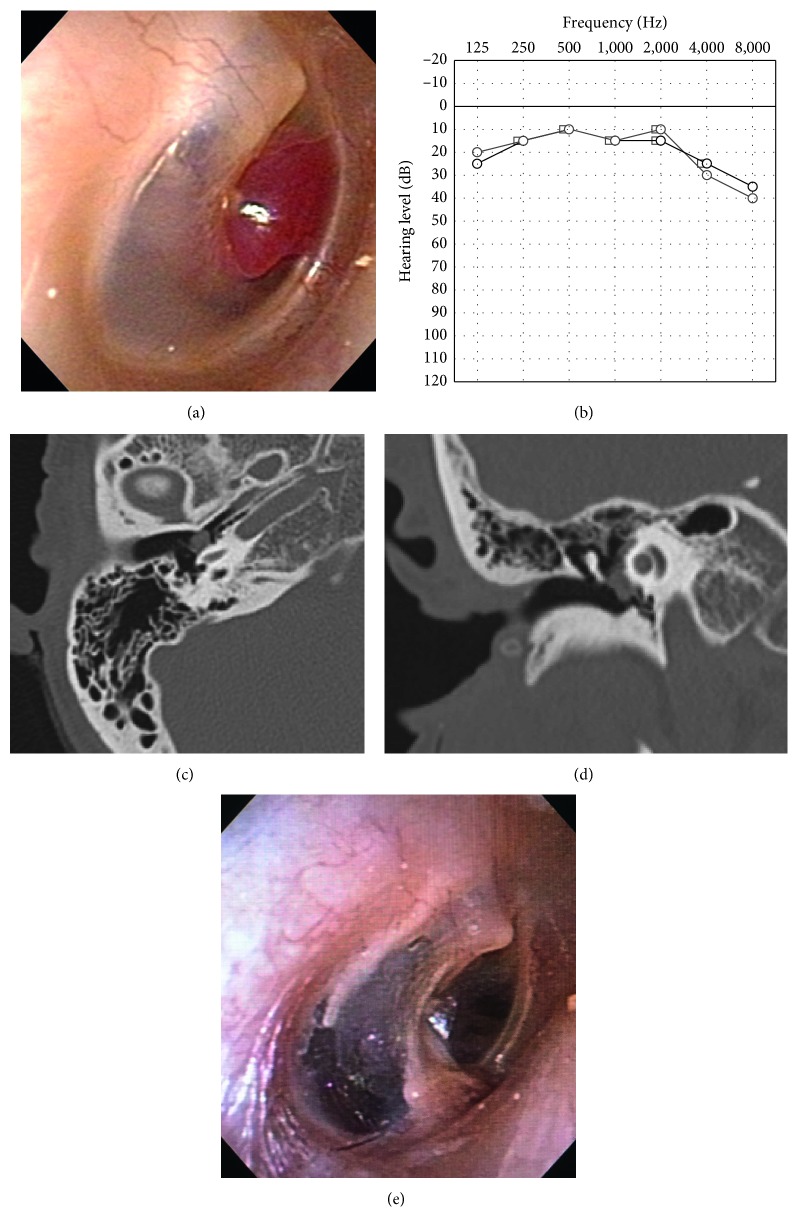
(a) A red mass was observed under the anterior quadrant of the tympanic membrane. (b) Pure-tone audiometry. The black and gray lines show the preoperative and postoperative hearing levels, respectively, in the right ear. After the surgery, the hearing level was not reduced and a normal hearing level was preserved. (c, d) A high-resolution computed tomography scan ((c) axial view and (d) coronal view) shows a mass on the promontory. (e) Postoperative tympanic membrane.
